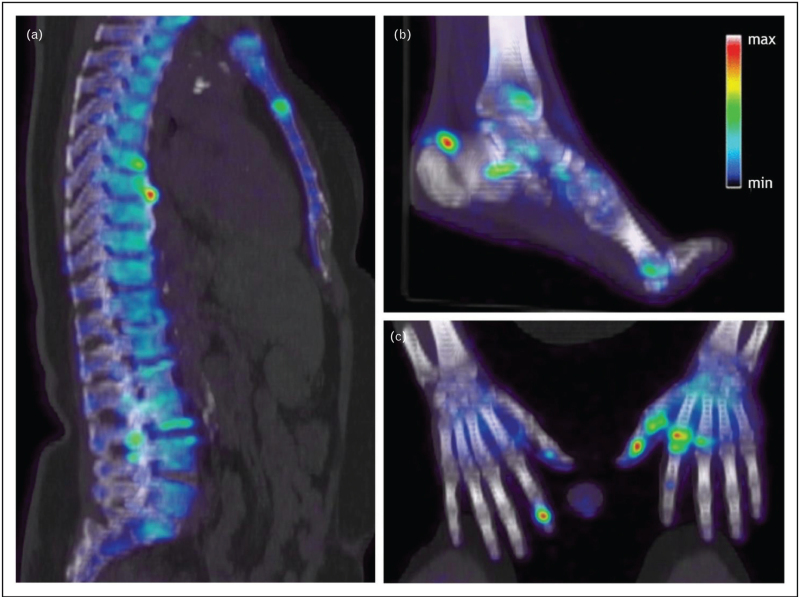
NaF uptake on PET/computed tomography in psoriatic arthritis patients. Figure 1 shows NaF enhancement in the axial spine (a), at the Achilles tendon insertion (b) and in the distal interphalangeal and metacarpophalangeal joints of the hands (c) in psoriatic arthritis patients.
